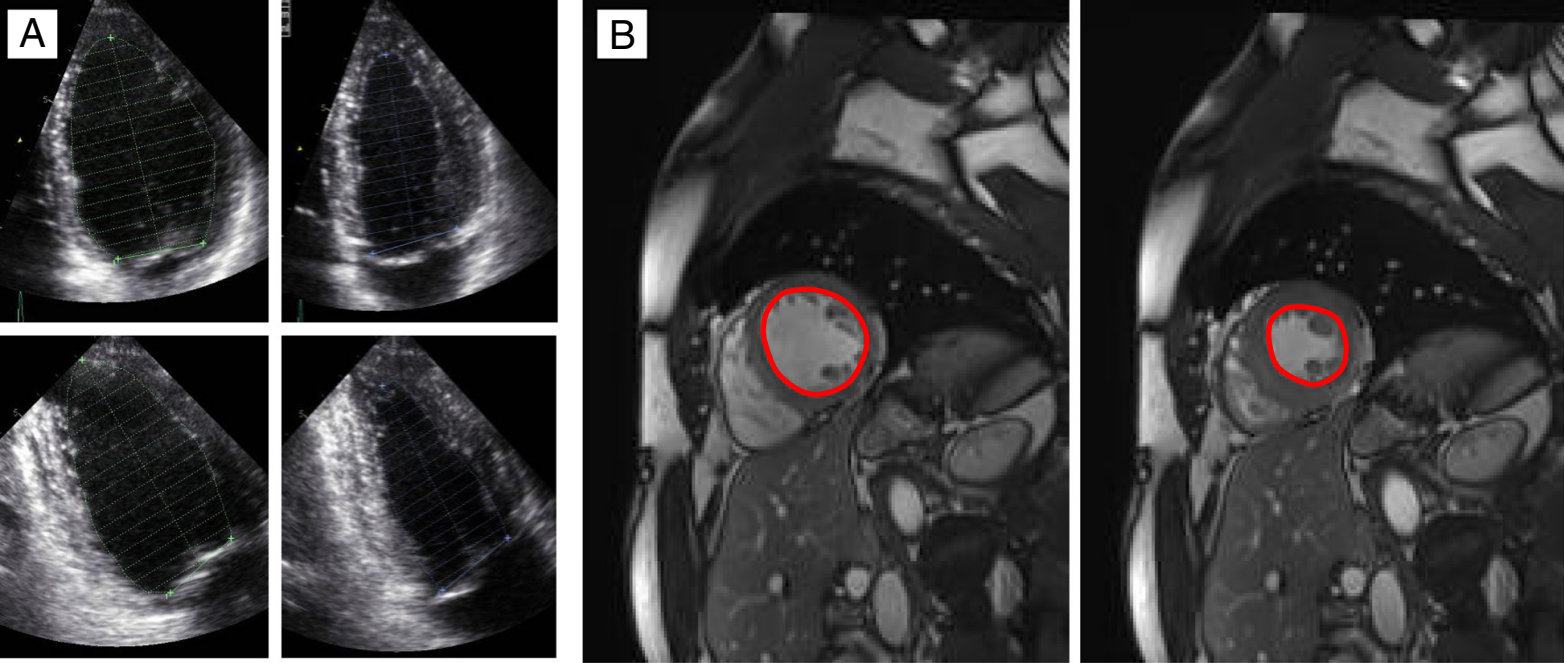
Measurement of the ejection fraction by 2D TTE and CMR. A. The LVEF by 2D TTE was obtained by the modified Simpson’s method in the apical four- and two-chamber view. B. The LVEF by CMR was calculated by assessment of end-diastolic and end-systolic LV volumes in multiple parallel short-axis slices.
